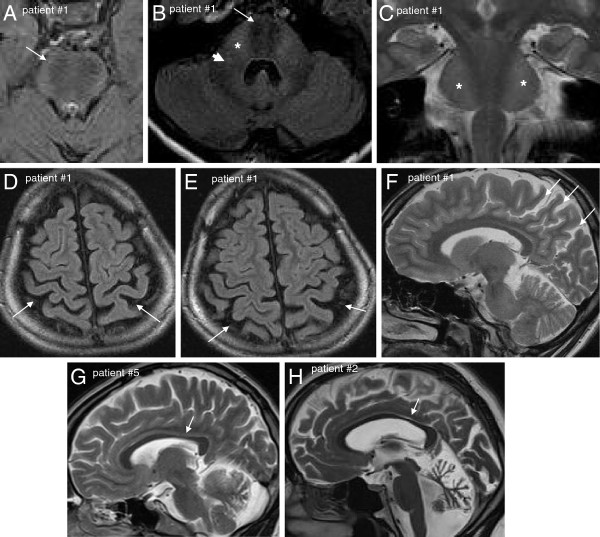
Characteristic MRI findings in ARSACS. Axial FLAIR images of upper pons (A) and middle pons (B) show hypointense strips in the central pons representing the cortico-spinal tract (white arrows): Moreover, on the level of the mid-pons (B), they reveal diffuse slight hyperintensity of the lateral pons when merging into the middle cerebellar peduncles (stars) and thickened middle cerebellar peduncles (white arrow head) in patient #1. Also on coronal T2 images (C) the bilateral hyperintensity of the lateral pons can be seen (stars). Bilateral post-central and parietal atrophy is shown for the same patient in axial FLAIR (D, E) and sagittal T2 (F) images (white arrows). Thinning of the posterior mid-body of the corpus callosum on sagittal T2 images is shown for patient #5 (G) and patient #2 (H) (white arrows).
